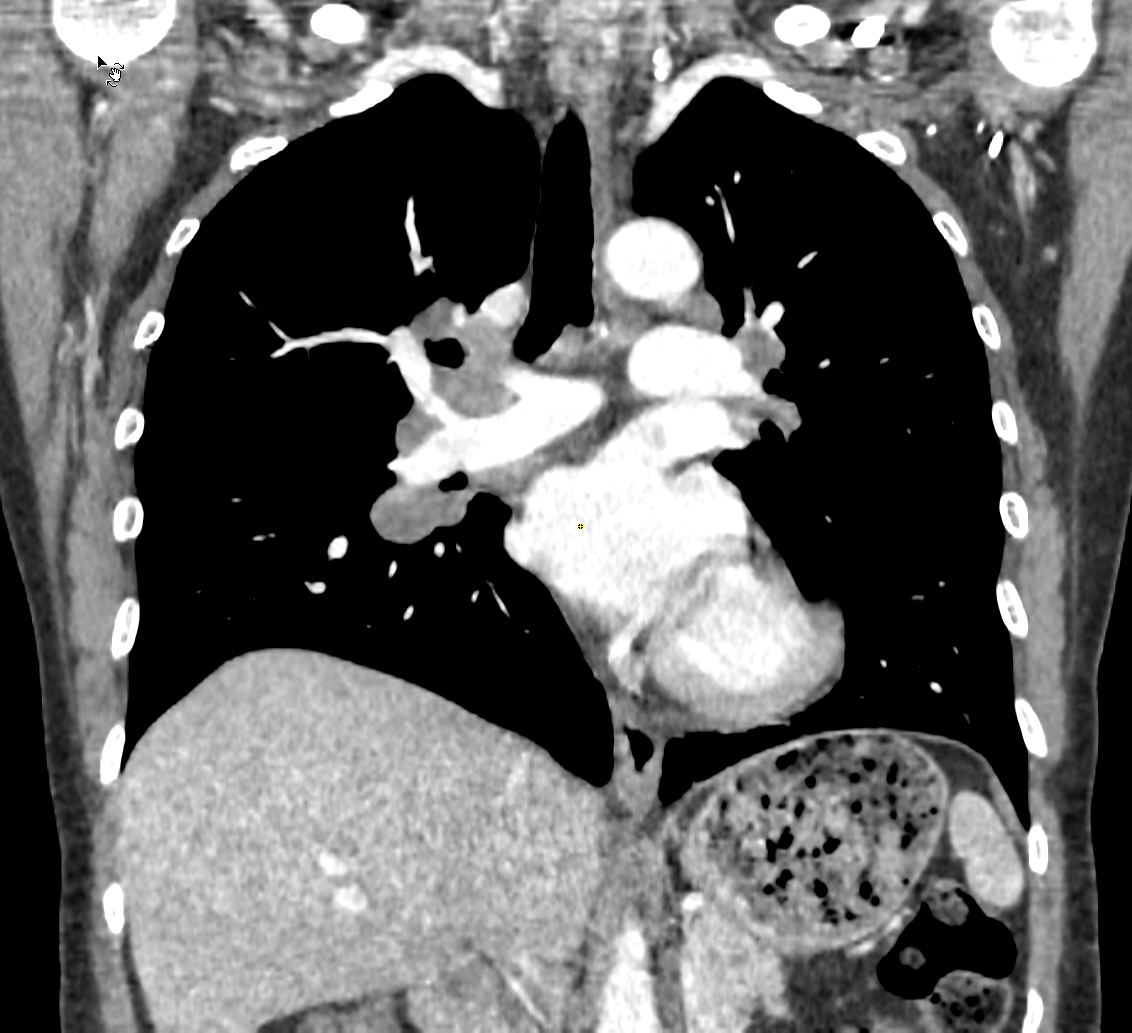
CT (2017) of the chest and upper abdomen showing mediastinal enlarged lymph nodes
